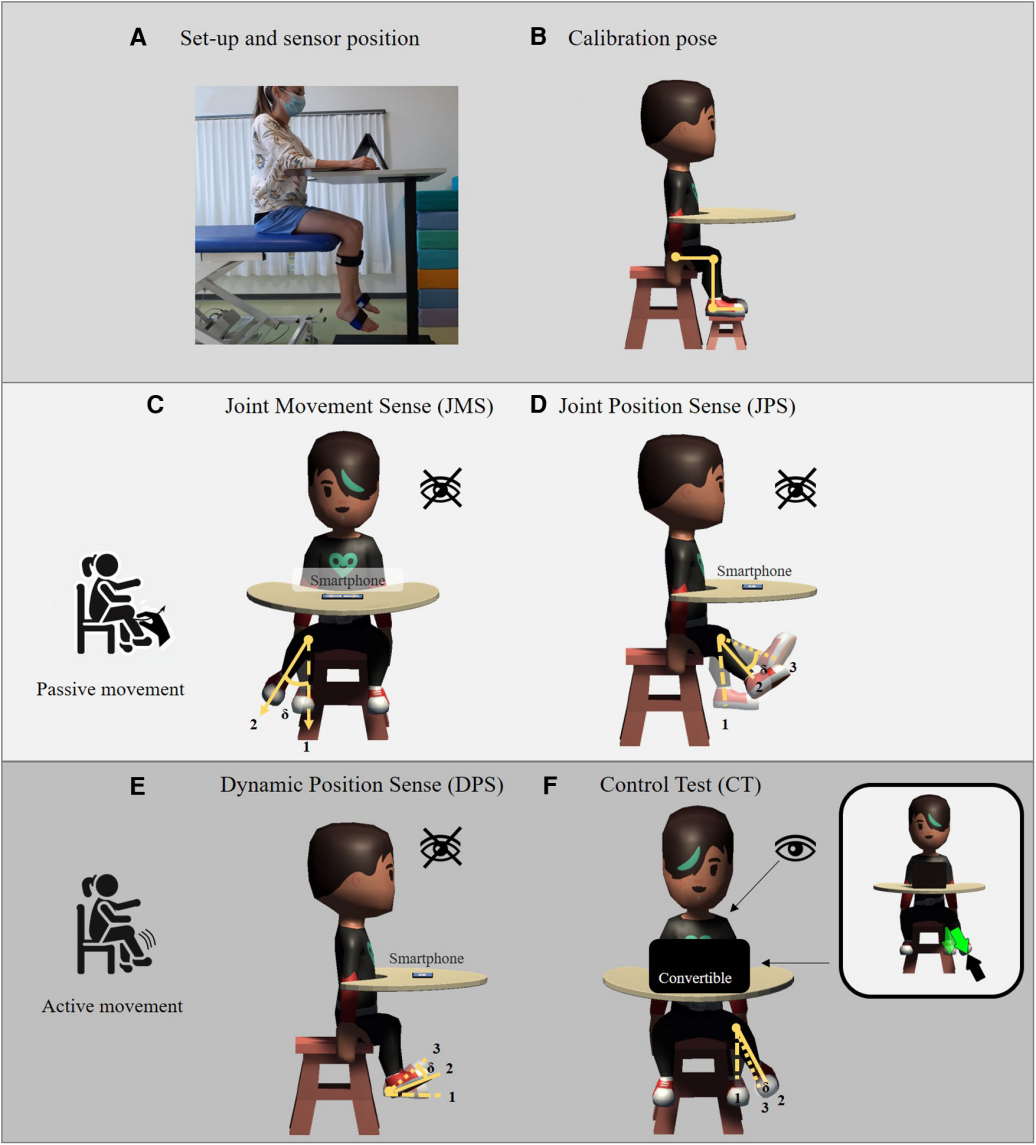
Test setting and modalities of the proprioception measurement tool (ProMeTo). (A) Set-up with a U-table preventing the child from seeing his or her legs, and sensor position at the calf and foot with neoprene cuffs; (B) Calibration in 90° hip flexion, 90° knee flexion, 90° dorsiflexion, and neutral for rotation and abduction represented in the avatar that guided the assessor through the tests (C) Joint movement sense (JMS), the rater moved the leg of the child; (D) Joint position sense (JPS), the rater moved the leg of the child; (E) Dynamic position sense (DS), the child actively moved the leg toward the criterion position, and (F) Control test (CT) where the child had visual feedback showing errors on the convertible notebook. 1 = starting position; 2 = confirmed position of the child; 3 = criterion position; *δ* = difference of the angle between the confirmed and the criterion position.
